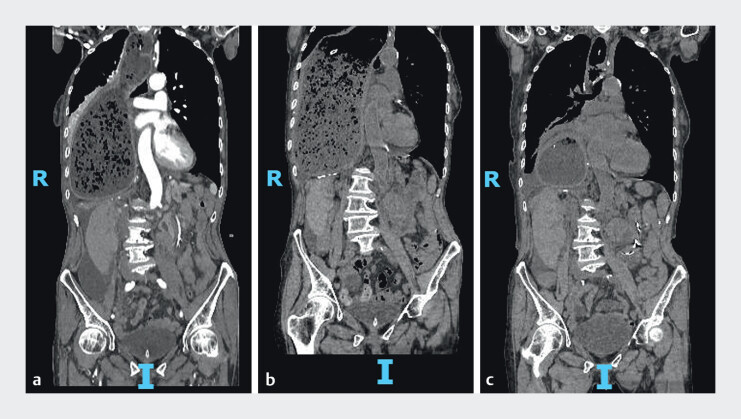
Computed tomography images obtained at the same coronal level.
